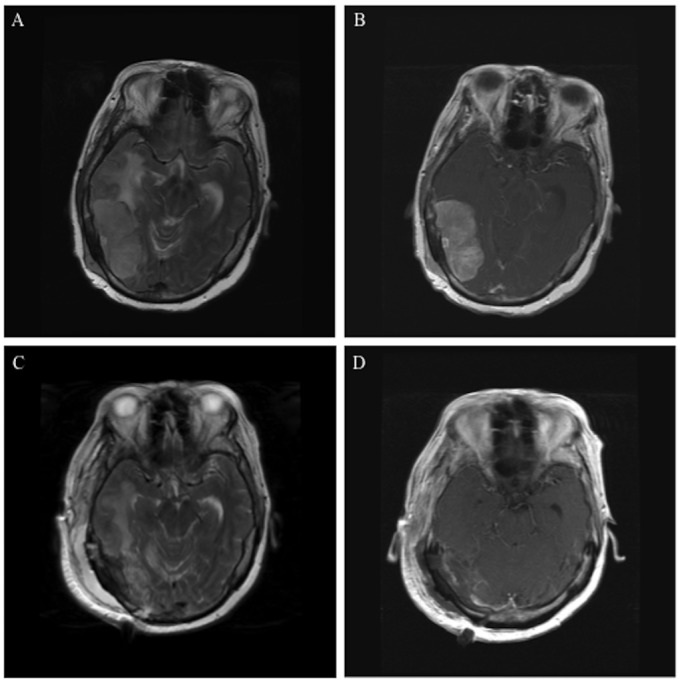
Resection of Dural-based Brain Metastasis Pre- and postoperative MRI images demonstrate resection of a breast cancer dural-based metastasis. Preoperative axial T2 (A) and T1 with contrast images (B) show a heterogeneously enhancing 2.9 x 5.9 x 2.9 cm dural-based tumor in the right temporal-parietal region associated with significant peri-tumoral edema and midline shift. Postoperative T2 (C) and T1 with contrast images (D) demonstrate tumor resection.
